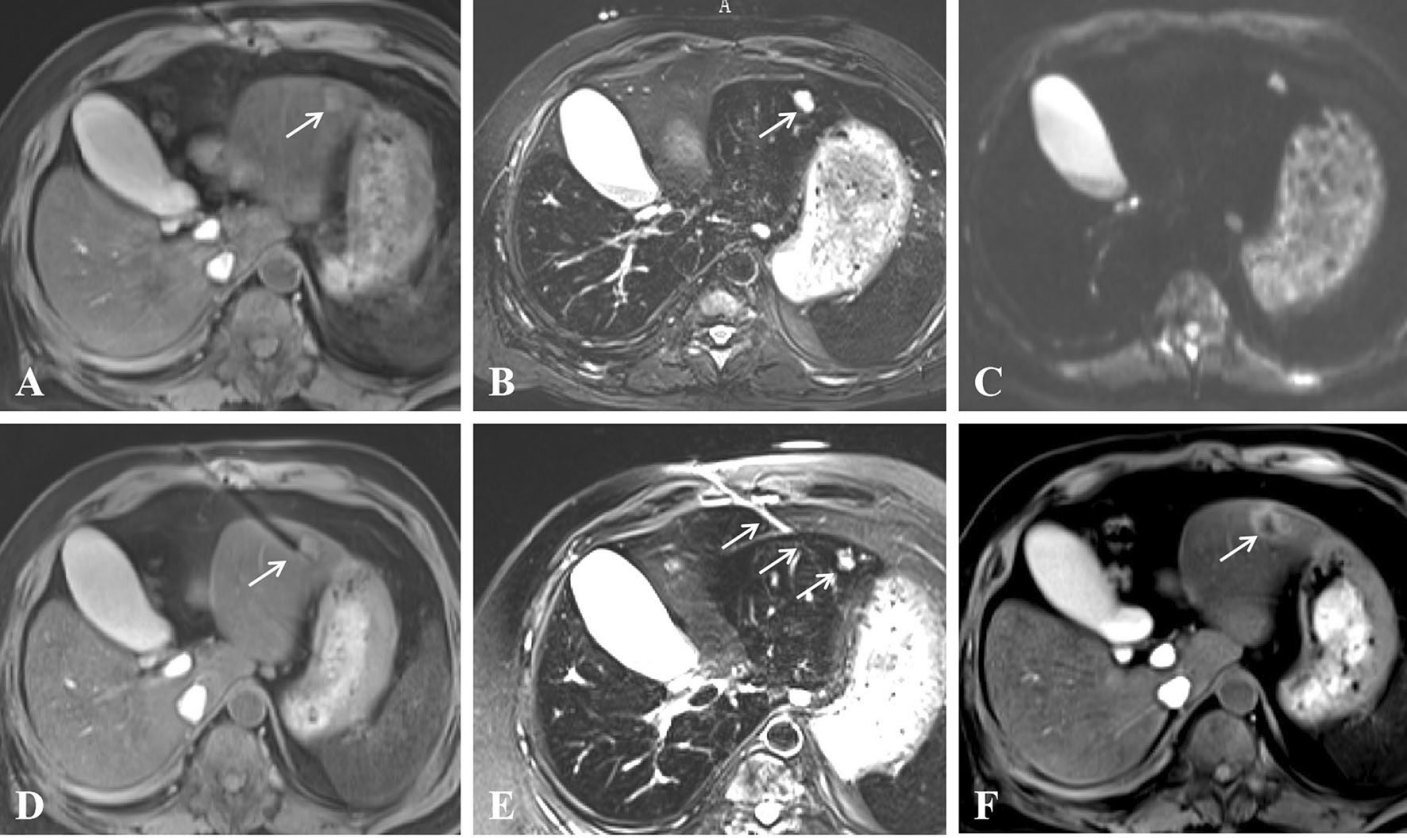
A 66-year-old man with liver metastasis at segment 3 (primary diagnosis was rectal carcinoma and underwent surgical resection). (A–C) Pretreatment MR showed a small lesion (diameter = 1.3 cm) on T1WI (A), T2WI (B) and DWI (C); (D,E) Lesion was punctured by a microwave applicator on T1WI (D) and T2WI (E), and high signal exudation associated with puncture directed by white arrows on Figure (E); (F) T1WI showed that the ablation focus was ellipsoidal with a high signal, which means complete ablation.
